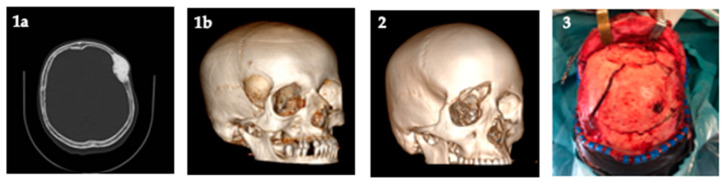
Example of causes that require cranioplasty (authors personal database images): (1a) axial computerized tomography (CT) scan of tumor involving cranial bone; (1b) 3D-CT scan of tumor involving cranial bone; (2) 3D-CT scan of depressed skull fracture; (3) surgical exposition of complex depressed skull fracture.
